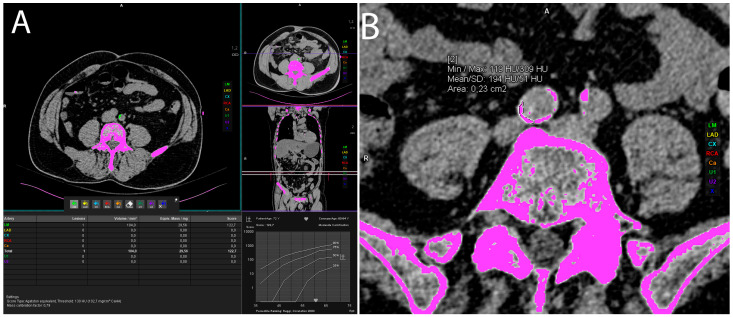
Representative axial CT images showing vascular calcification measurement by manually labeling every calcification in the artery of interest (A) or using freehand ROI method in case of larger calcifications (B).
